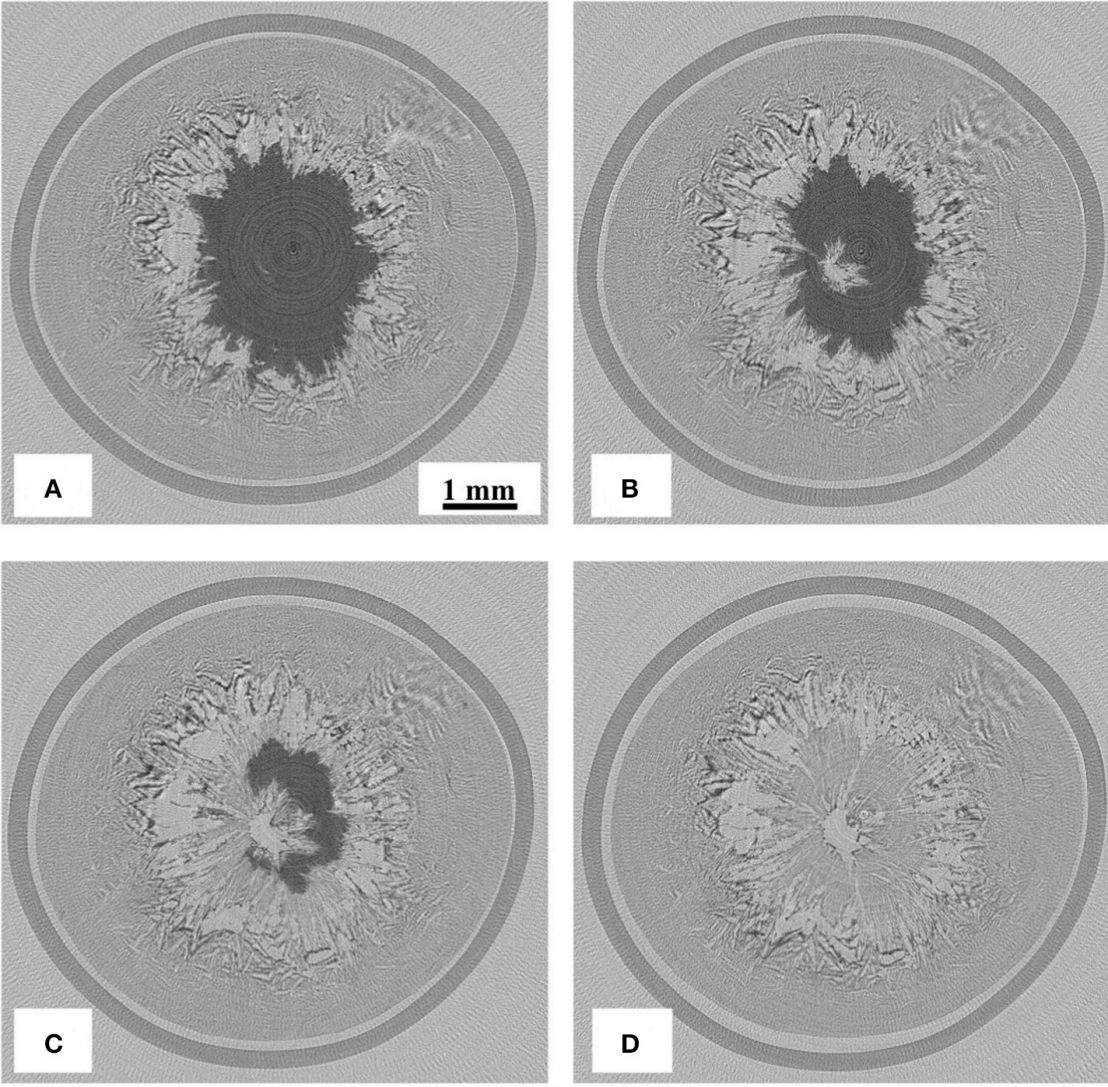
Two-dimensional (2D) X-ray CT images during freeze-drying of the non-annealed samples (horizontal cross-section at 900 μm from the top), dried for (A) 1,800, (B) 2,400, (C) 3,000, and (D) 3,900 s.
